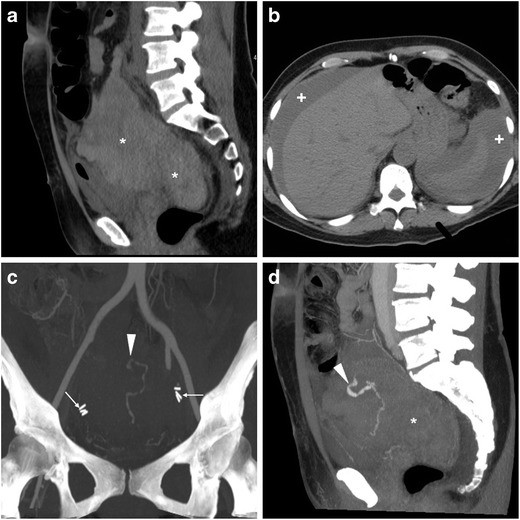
Haemoperitoneum after LH for uterine myomas, manifesting the next day with worsening pelvic pain and blood loss. Precontrast CT (A, B) images showed haemoperitoneum (+) in the upper abdomen, and a vast hyperattenuating haematoma (*) occupying most of the pelvis, causing compression on the urinary bladder. CT angiography (C) and venous-phase CT acquisition (D) showed active arterial bleeding as serpiginous contrast medium extravasation (arrowheads). Open surgery confirmed presence of nearly 1 l of blood in the abdominal cavity and arterial haemorrhage from the left-sided angle of the vaginal vault and small vessels of the ipsilateral ovarian pedicle, which was treated by coagulation and suture (Partially reproduced from open access ref. no. [60])
